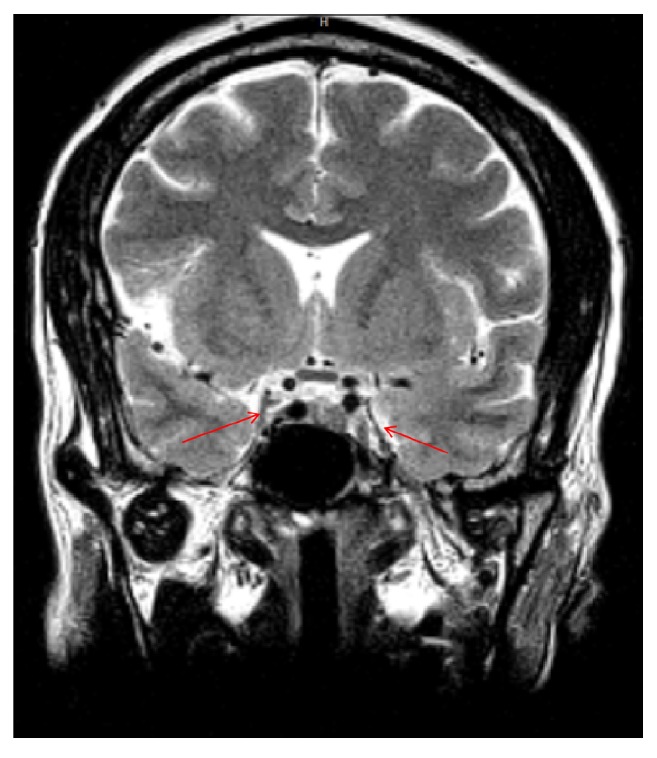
Coronal T1 diffusion-weighted MRI of the pituitary gland.
